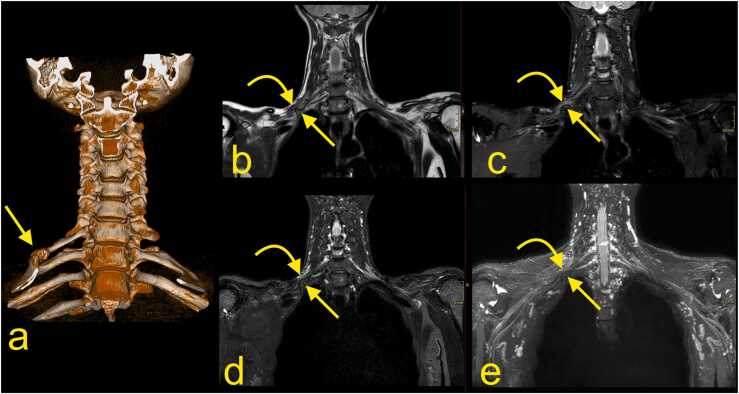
A 42-year-old patient with an accessory cervical rib on the right side shown in (a) CT with 3D reconstruction (straight arrow) was detected one year earlier because of pain on the right side of the neck when lying down and during specific movements. The patient now presented with weakness of the right-hand muscles and occasional paresthesia in the right arm. Clinically, thoracic outlet syndrome was suspected. MRI with (b) T2-weighted DIXON TSE, (c, d) T2-weighted DIXON TSE with fat suppression, and (e) MR neurography showed that the accessory rib (straight arrow) dislocated and distorted the lower trunk of the BP (curved arrow).
